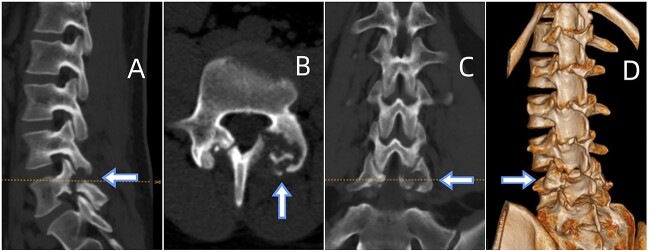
(A) Sagittal CT displaying lumbar spondylolysis at L5. (B) Axial CT revealing articular subchondral erosions and soft tissue masses at the left facet joint L5-S1. (C) Coronal CT and (D) 3D image demonstrating articular erosions and punched-out lesions of L5-S1, along with spondylolysis at L5.
